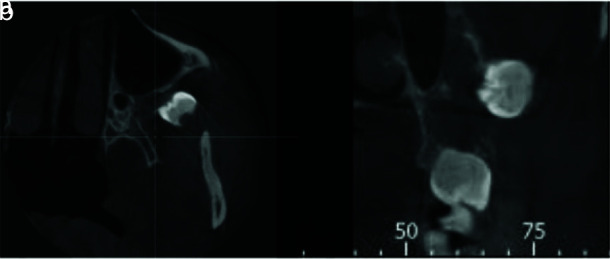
a: The axial view of a post-complication CT scan revealed the new position of the third molar in the buccal space. b: The para-axial cuts of the CT scan showing the movement in the buccal space of the tooth
